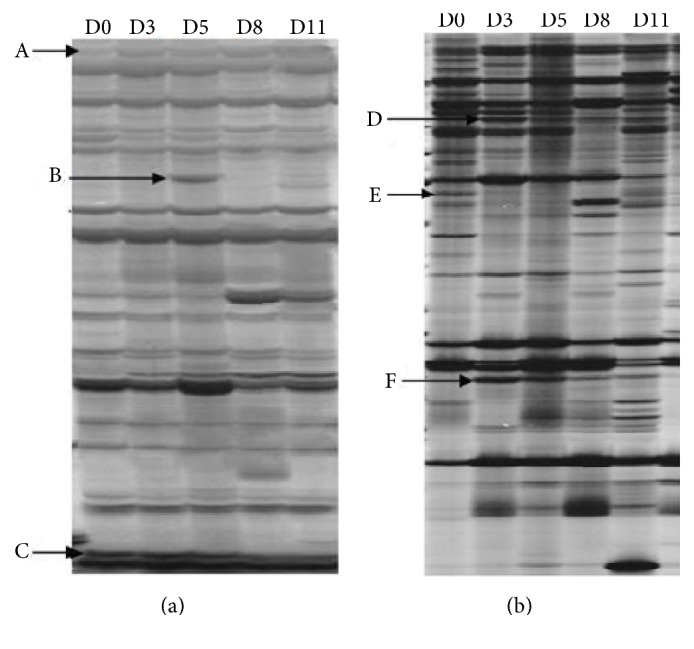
An example of cDNA-AFLP profile on stress-treated tangor. D0~D11: 0, 3, 5, 8, and 11 days after drought stress treatment, respectively; (a) and (b) cDNA-AFLP profile with AseI-CT/TaqI-GG and AseI-AT/TaqI-TC primer combination. The differentially expressed TDFs showed four expression patterns. A: upregulation; B, D, and F: induction; C: downregulation; E: repression. The TDFs of genes induced were present in drought stress but not in control. The TDFs of genes repressed were present in control but not in drought stress. The expression of upregulated and downregulated genes could be detected in both control and drought stress, with increased and decreased expression intensity under drought stress, respectively, when compared with control.
